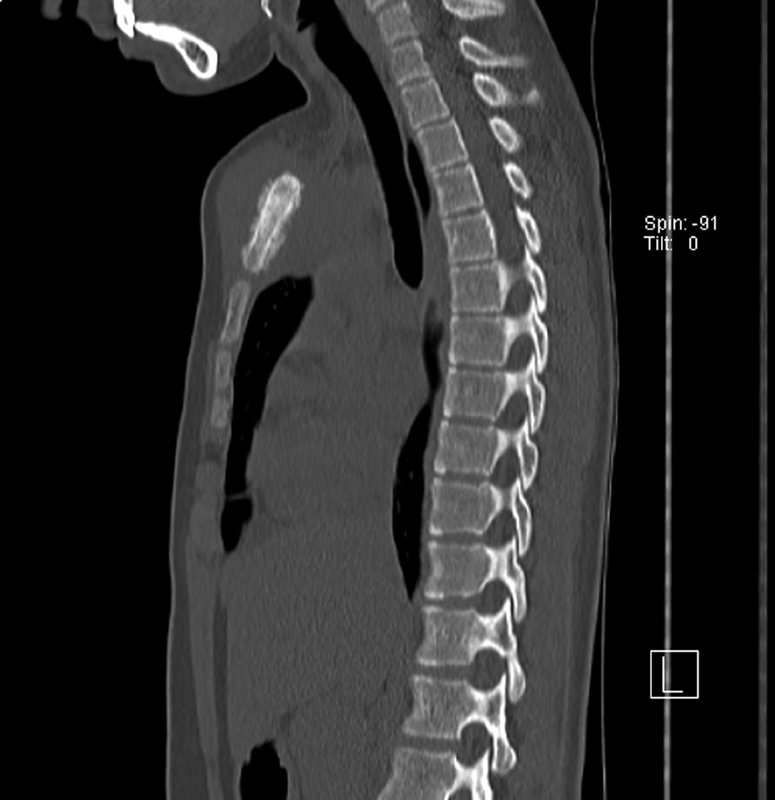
Preoperative computed tomography scan view of the sternum tumor.
